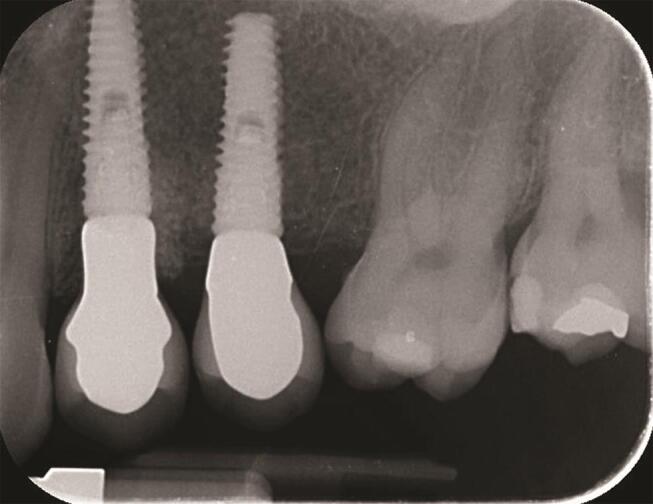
Periapical radiograph at the 1-year follow-up.
